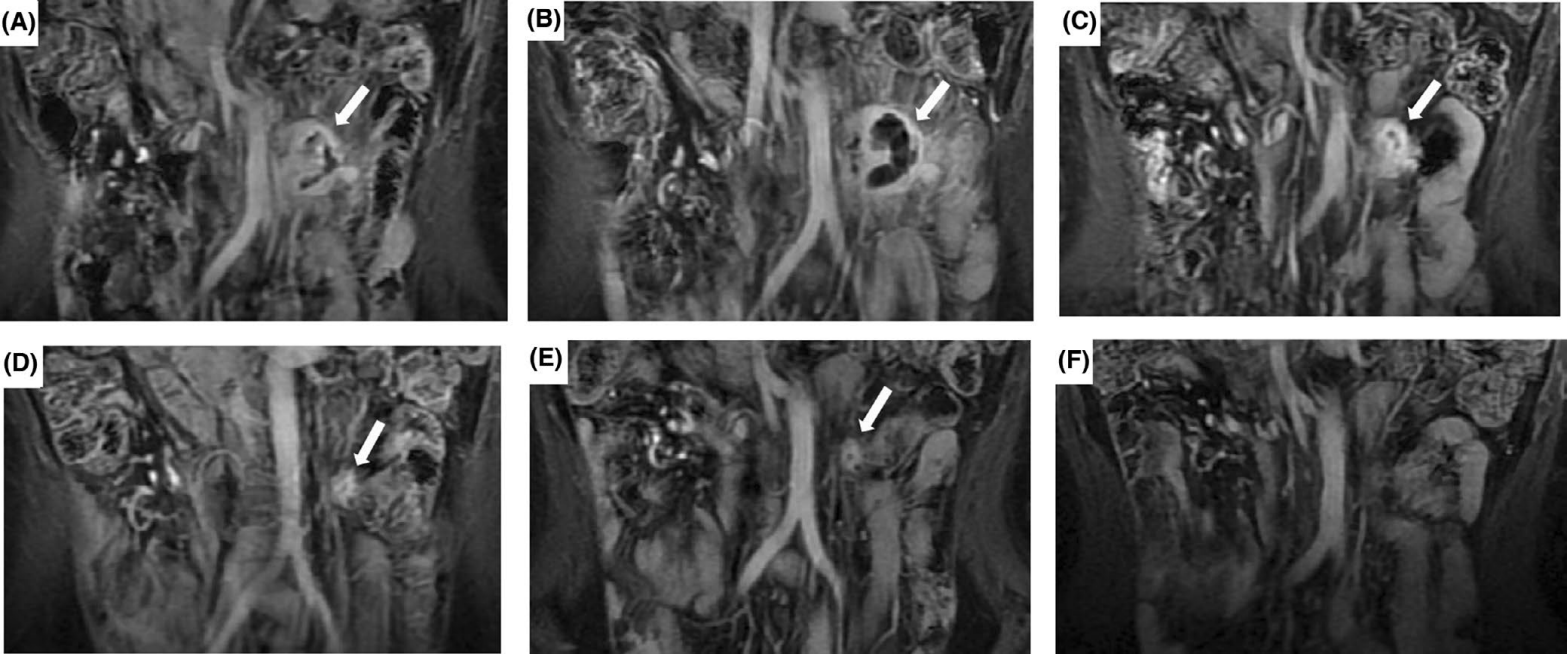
A recurrent desmoid tumor in a 46‐year‐old woman was carefully evaluated by MRI. (A). MRI images show intra‐abdominal recurrence before HIFU treatment; (B). Significant necrosis can be observed after 3 days; (C). Tumor shrinkage can be observed after 5 months; (D). MRI images after 12 months; (E). MRI images after 20 months; (F). The complete remission of the recurrent desmoid tumor can be observed after 41 months of follow‐up after HIFU treatment. MRI, magnetic resonance imaging; HIFU, high‐intensity focused ultrasound
